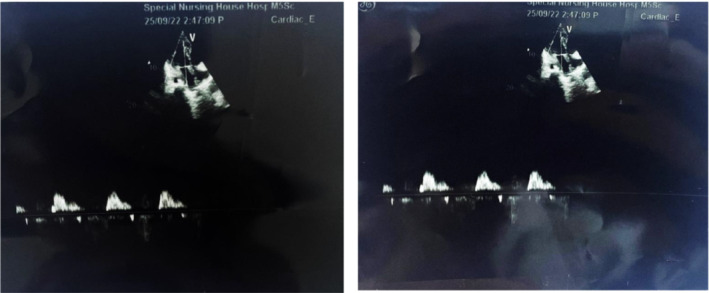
Echocardiography of the patient.
